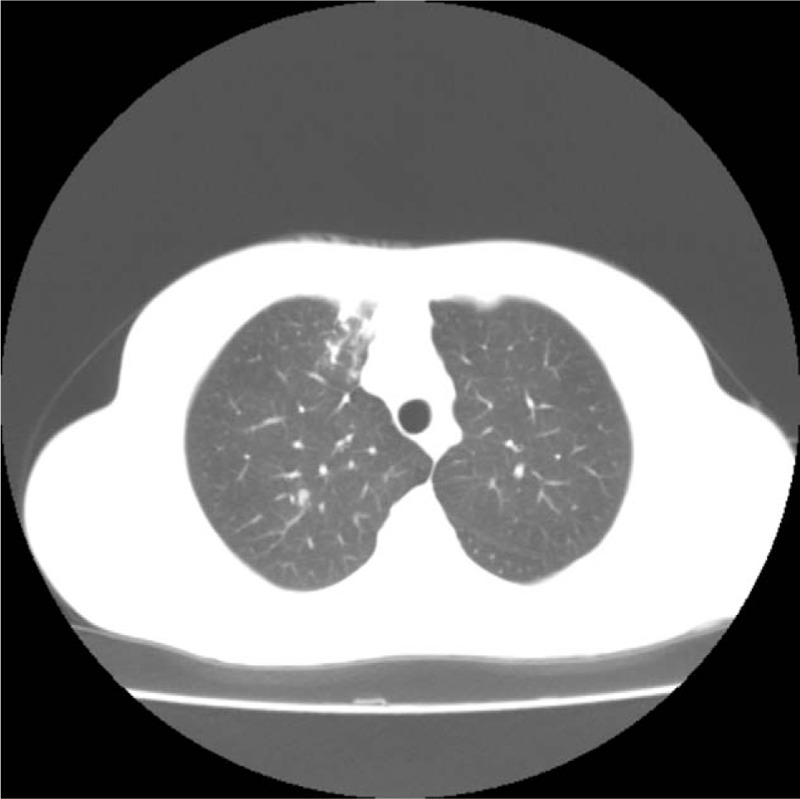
Chest computed tomography revealed evidence of infectious disease in the right upper lung lobe and enlargement of a mediastinal lymph node.
